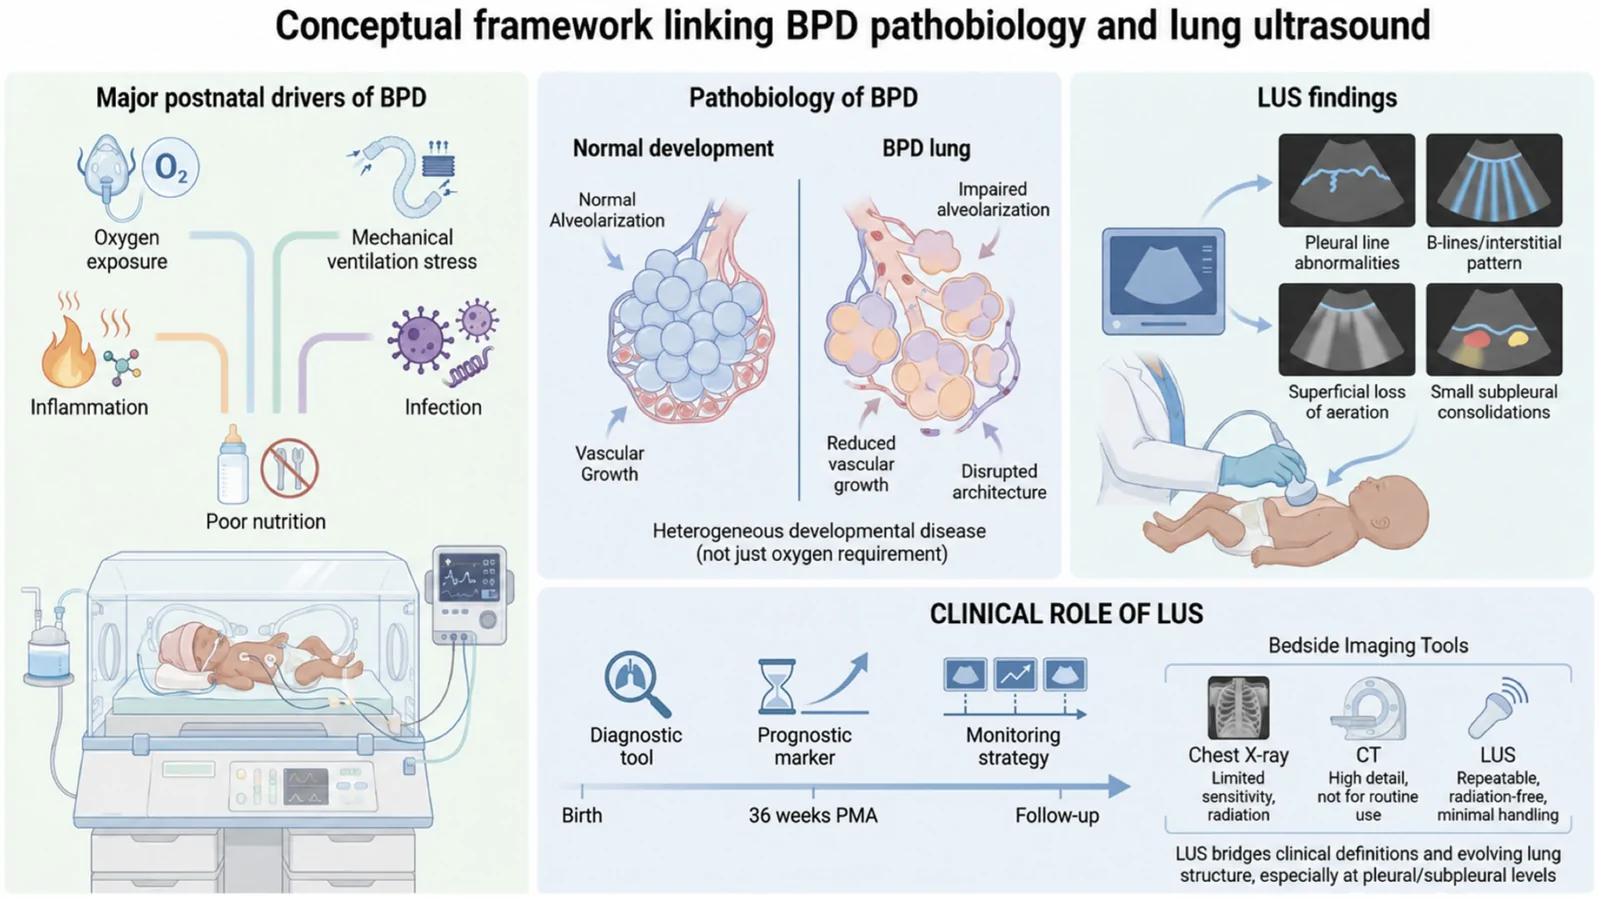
A conceptual framework linking the pathobiology of BPD with the clinical application of LUS. Prematurity-associated postnatal insults, including oxygen exposure, mechanical ventilation, inflammation, infection, and poor nutrition, disrupt normal alveolar and pulmonary vascular development, leading to the heterogeneous phenotype of modern BPD. Because LUS interrogates the pleural surface and adjacent subpleural lung, it identifies indirect sonographic signs of peripheral lung injury, including pleural line abnormalities, vertical artifacts (B-lines), superficial loss of aeration, and small subpleural consolidations. These characteristics support the use of LUS as a bedside imaging modality for bedside assessment, early risk stratification, and longitudinal monitoring throughout the neonatal course. BPD, bronchopulmonary dysplasia; CT, computed tomography; LUS, lung ultrasound; PMA, postmenstrual age. Figure created using FigureLabs https://www.figurelabs.ai/ accessed on 10 July 2026.
